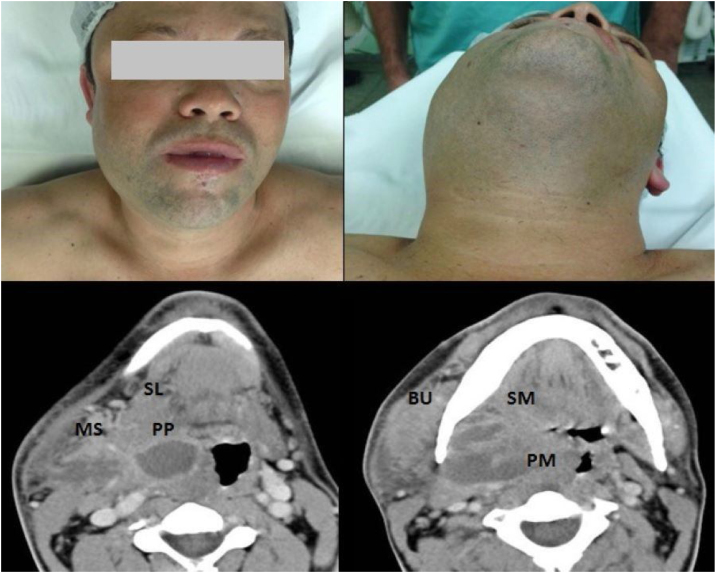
Case # 2. A 49-year-old male smoker presented with an odontogenic infection of the right upper third molar and who was hospitalized for 14 days, including 4 days in the ICU. (a) Areas of swelling in the right hemiface; (b) Areas of swelling in the submandibular region; CT with contrast revealing a collection in the Parapharyngeal (PP) space extending to the Sublingual (SL) and Masseteric (MS) spaces; (d) Collection in the ipsilateral Pterygomandibular (PM), Submandibular (SM) and Buccal (BU) spaces, promoting medial displacement of the right palatal tonsil and luminal reduction of the oropharynx (e) Collection in the right Masseteric Space (MS).
